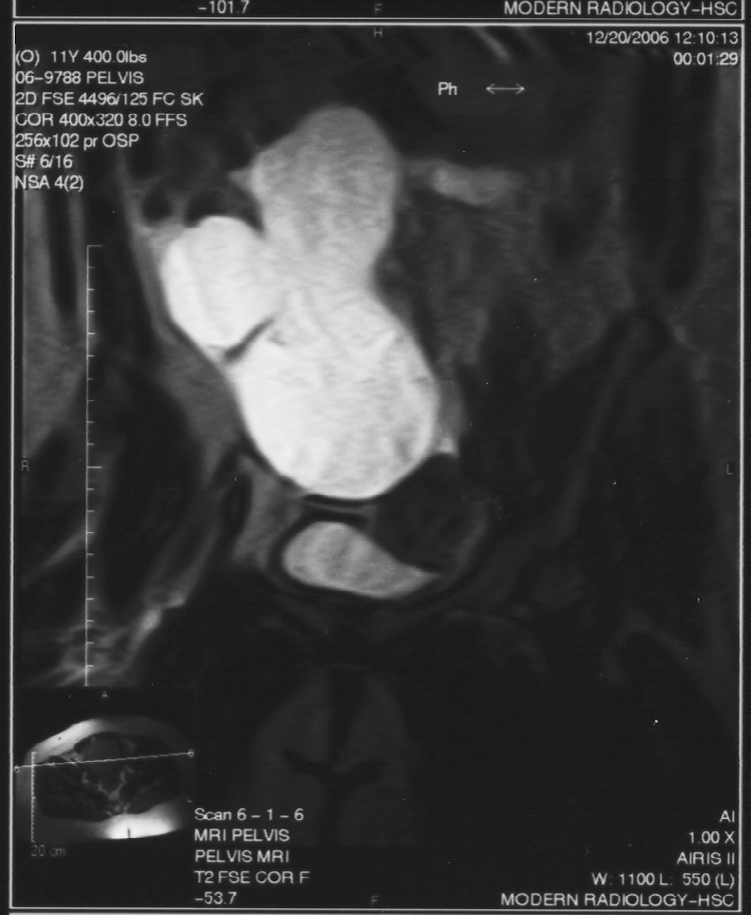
Coronal T2-weighted MRI.
